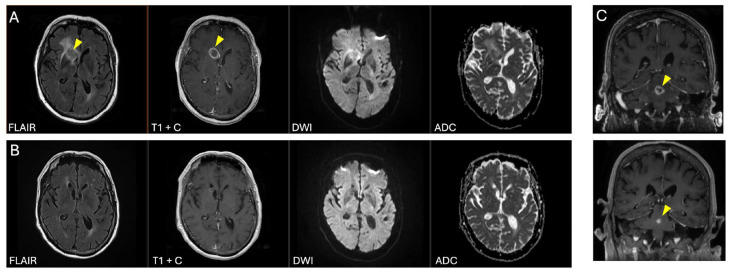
Axial and coronal MRI images demonstrating basal ganglia and pontine masses at sequential timepoints. (A) Axial images at initial presentation and (B) at surveillance entry showcasing basal ganglia mass (arrowhead). (C, top) Coronal MRI image at the time of mixed treatment response and (C, bottom) at surveillance entry with the pontine mass indicated (arrowhead). No diffusion restriction or microbleeds affected image quality. Abbreviations: FLAIR, Fluid-Attenuated Inversion Recovery; T1 + C, T1 with gadolinium contrast enhancement; DWI, Diffusion-Weighted Image; ADC, Apparent Diffusion Coefficient map.
